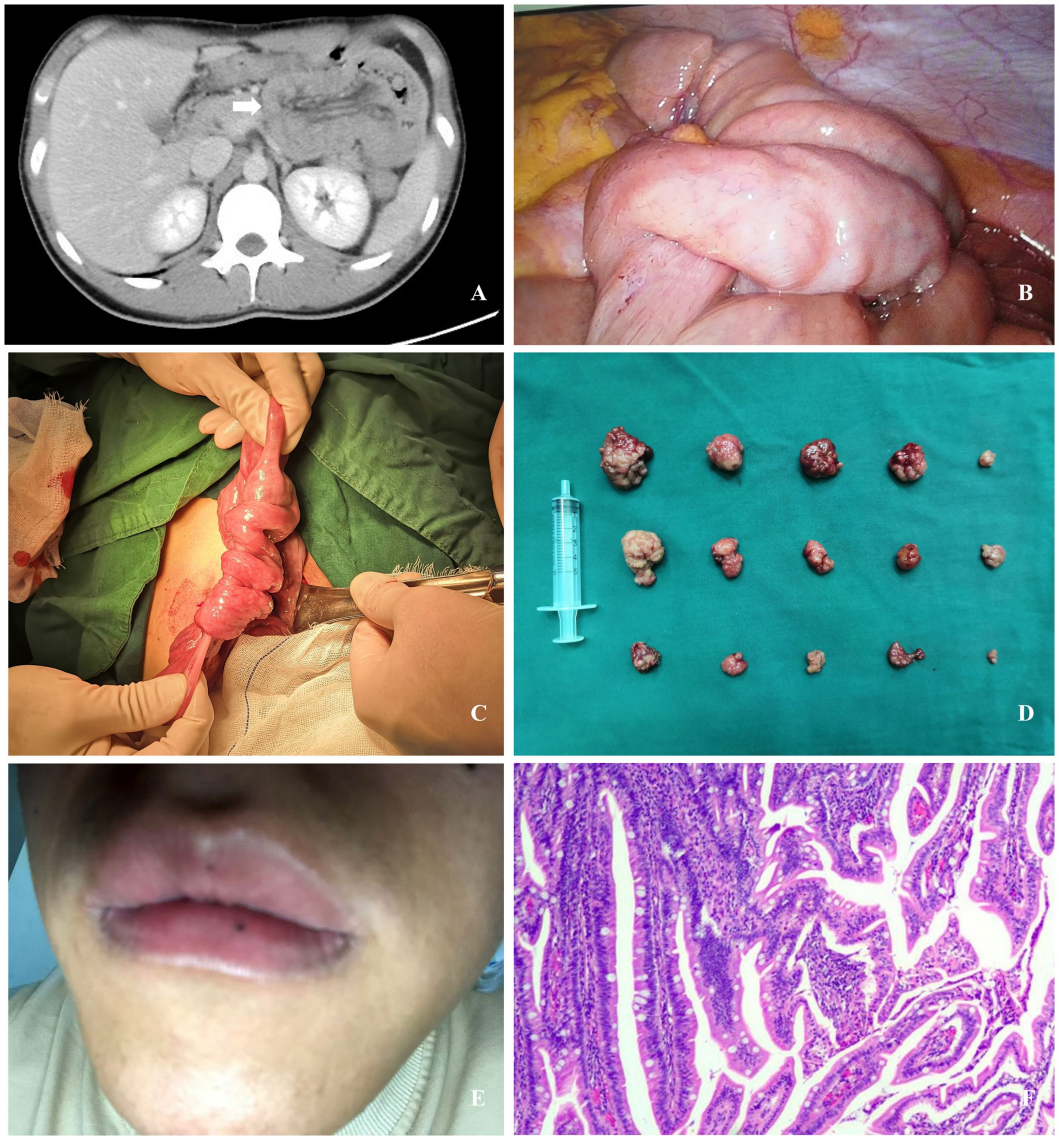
(A) Computed tomography revealed a “double-ring sign” in the intestinal loops within the left abdomen (white arrow). (B) Multiple instances of small intestinal intussusception were identified during laparoscopic exploration. (C) Small intestinal intussusception was identified during laparotomy. (D) Fifteen polyps were resected during the procedure. (E) A black macule measuring approximately 1.5 mm in diameter on the lower lip (the photograph was self-taken and provided by the patient post-discharge). (F) Postoperative pathology revealed hamartomatous polyps containing multiple small foci of low-grade intraepithelial neoplasia.
